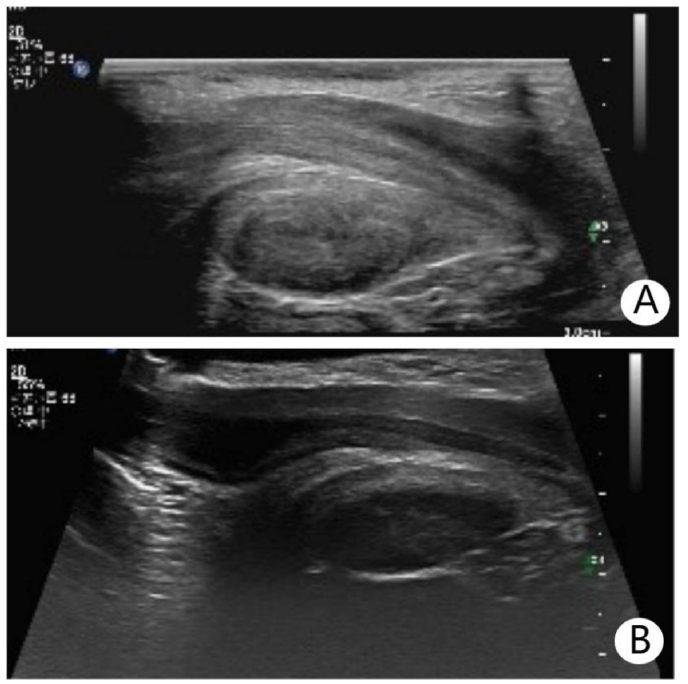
Follow-up color Doppler ultrasound images after completion of anti-inflammatory treatment. The imaging shows the urethra with a normal course, and no significant abnormal mass echoes are observed in the surrounding area.
